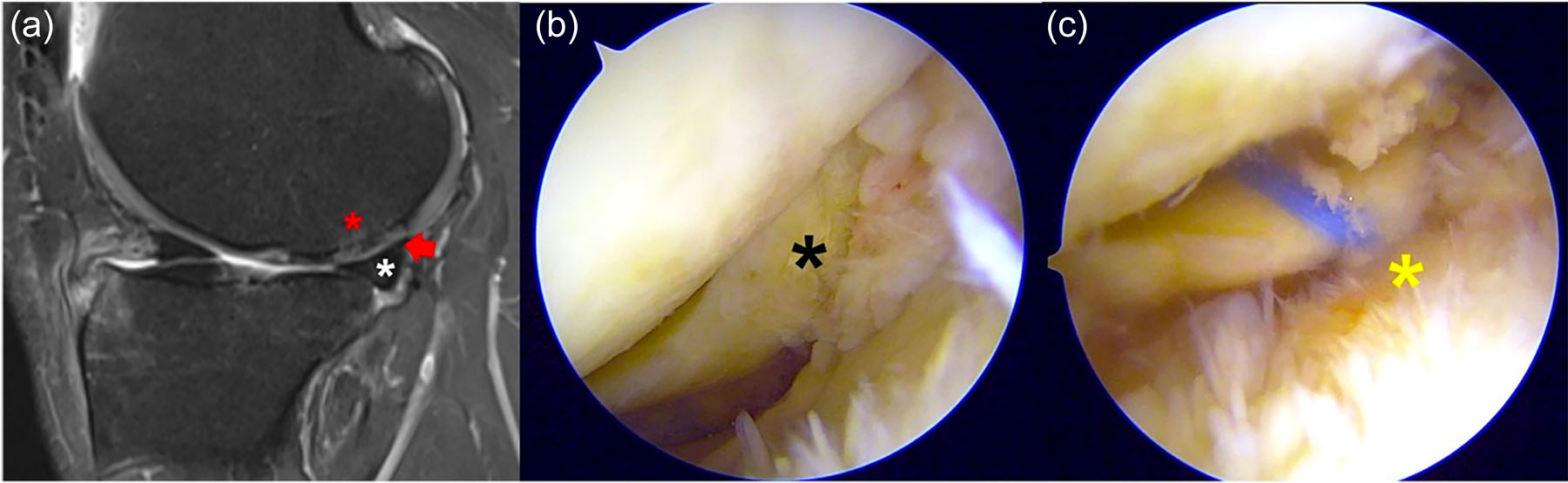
Right knee of a 39‐year‐old patient with chronic ACL tear. The sagittal MRI of the lateral compartment shows the presence of subchondral bone oedema (red asterisk) and cartilage thinning with tissue inhomogeneity and abnormal signal (red arrow) at the lateral femoral condyle, with an intact lateral meniscus (white asterisk) (a). At the time of arthroscopy, the posterior root (black asterisk) was torn from the tibial insertion and floating (b). The root (yellow asterisk) was repaired with a transtibial tunnel (c). ACL, anterior cruciate ligament; MRI, magnetic resonance imaging.
